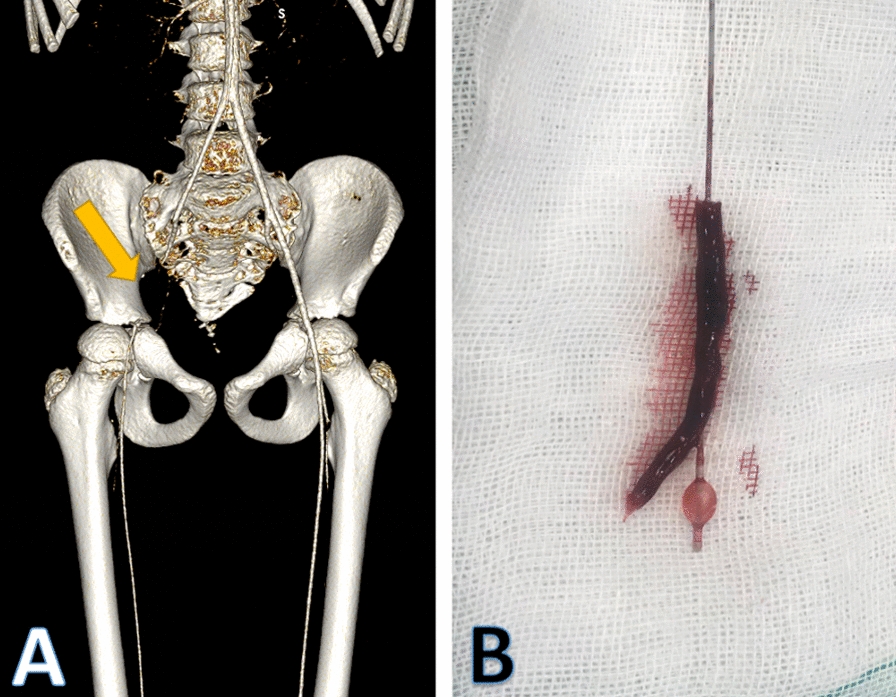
Images of the case of a 9-year-old male patient who underwent right femoral artery diagnostic catheterization before surgical repair of interventricular communication. a: Angio CT of iliac and femoral arteries, chondral spaces are appreciated in iliac bones, yellow arrow signaling right EIA thrombosis, b right EIA thrombus removed into the 2Fr Fogarty catheter
